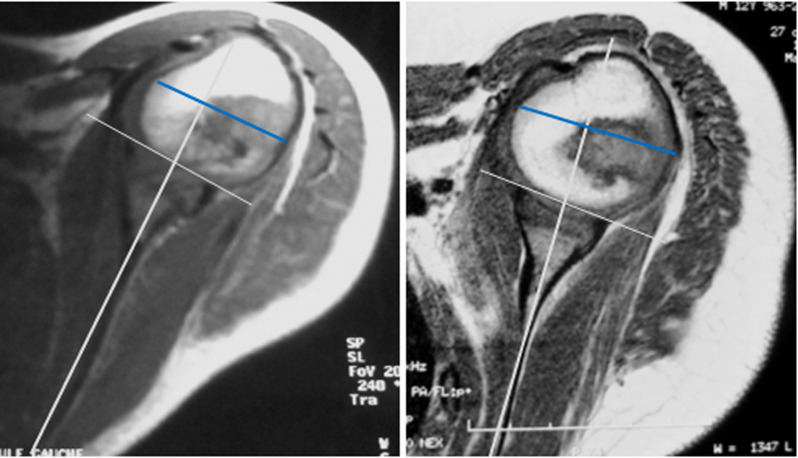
preoperative and 5 years postoperative MRI in a 5-year-old male with left brachial plexus palsy
